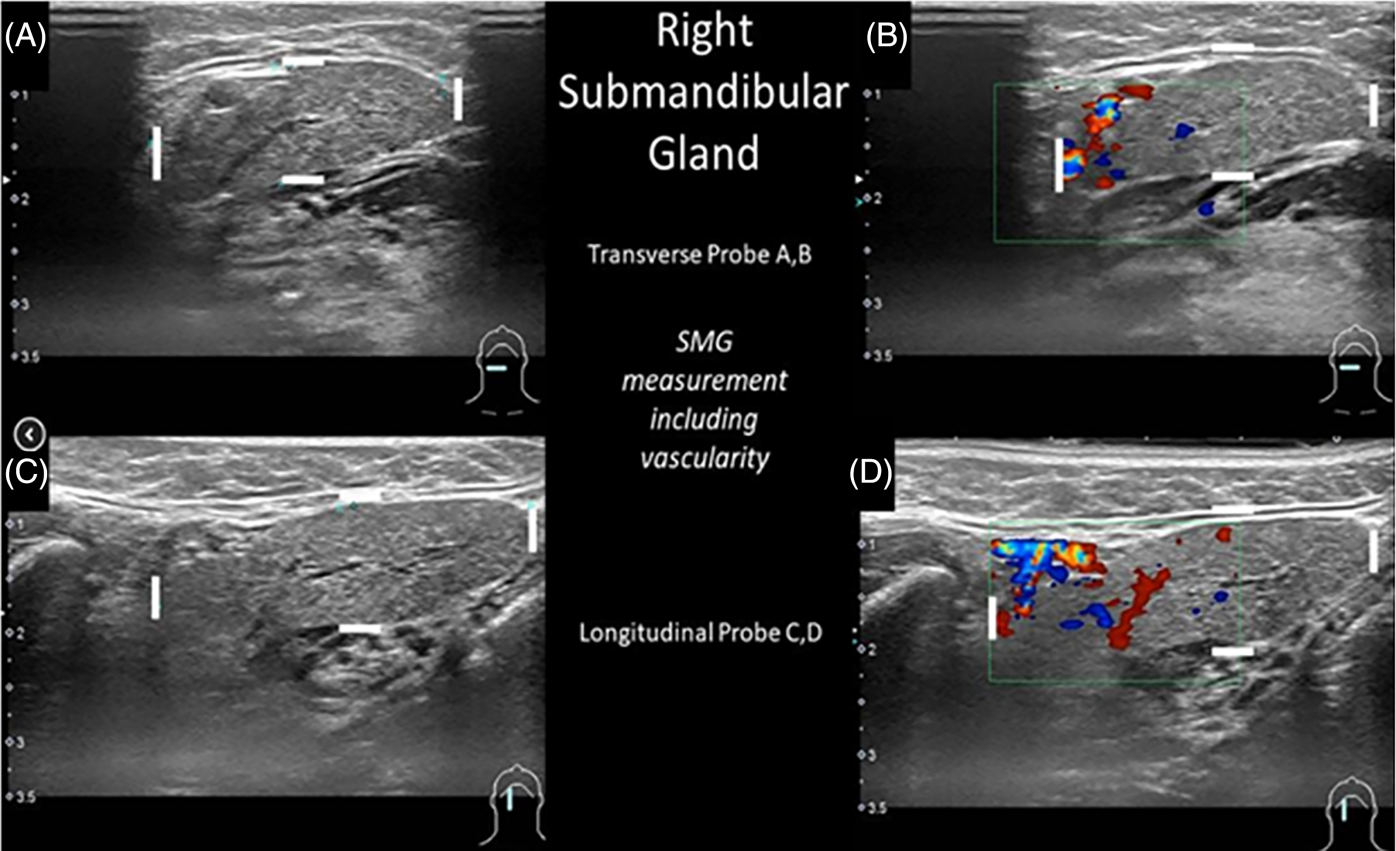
Ultrasound of right submandibular gland assessed with 5–14 MHz linear probe in transverse (3A, B) and longitudinal (3C, D) orientations identifying measurement of the size of the gland to include the blood vessels imaged with color doppler. (with approval from Hoffman HT (ed) Iowa Head and Neck Protocols <Submandibular Gland Anatomy: Vascular Supply—Ultrasound Imaging with Color Doppler https://medicine.uiowa.edu/iowaprotocols/submandibular‐gland‐anatomy‐vascular‐supply‐ultrasoundimaging‐color‐doppler> accessed June 18, 2023).
